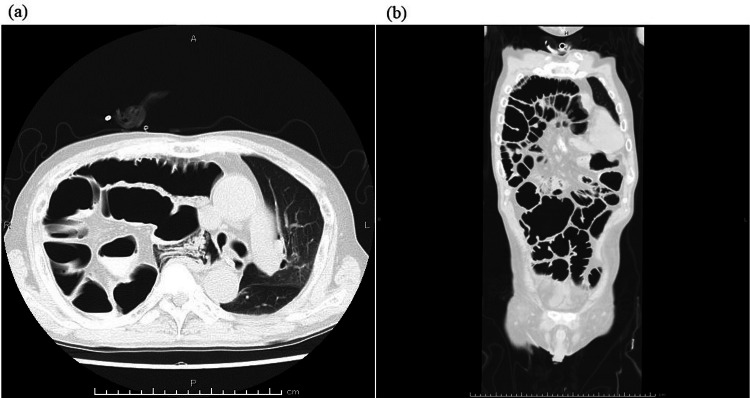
CT scan on POD 15. (a) The intestinal tract was fitted into the right thoracic cavity, resulting in a severely collapsed right lung and the mediastinum deviated to the left. (b) The right thoracic cavity was almost completely filled by the intestinal tract. CT, computed tomography; POD, postoperative day
